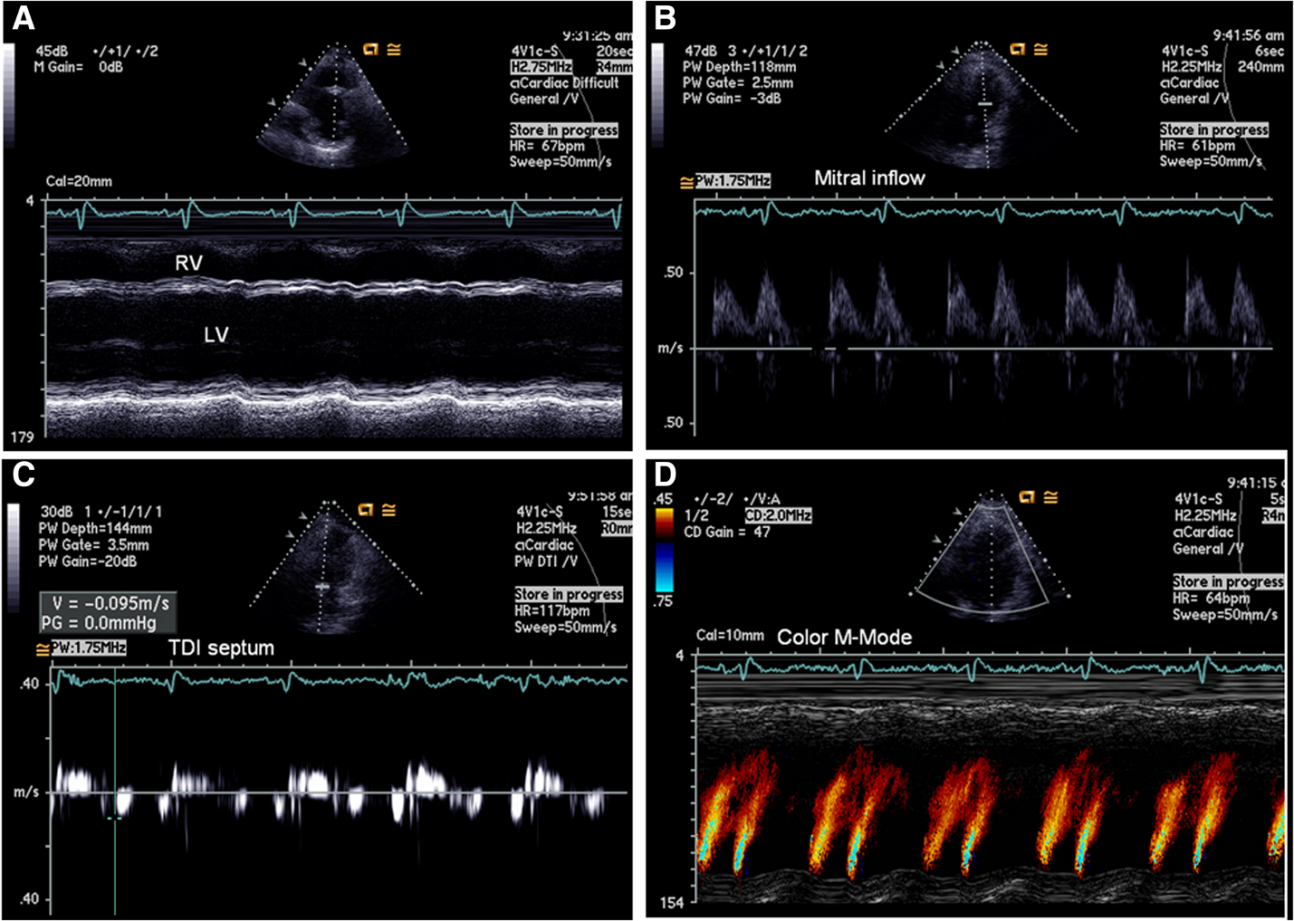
Echocardiographic images showing severe left ventricular systolic dysfunction (A) and a grade 2 diastolic dysfunction pattern (B to D).
